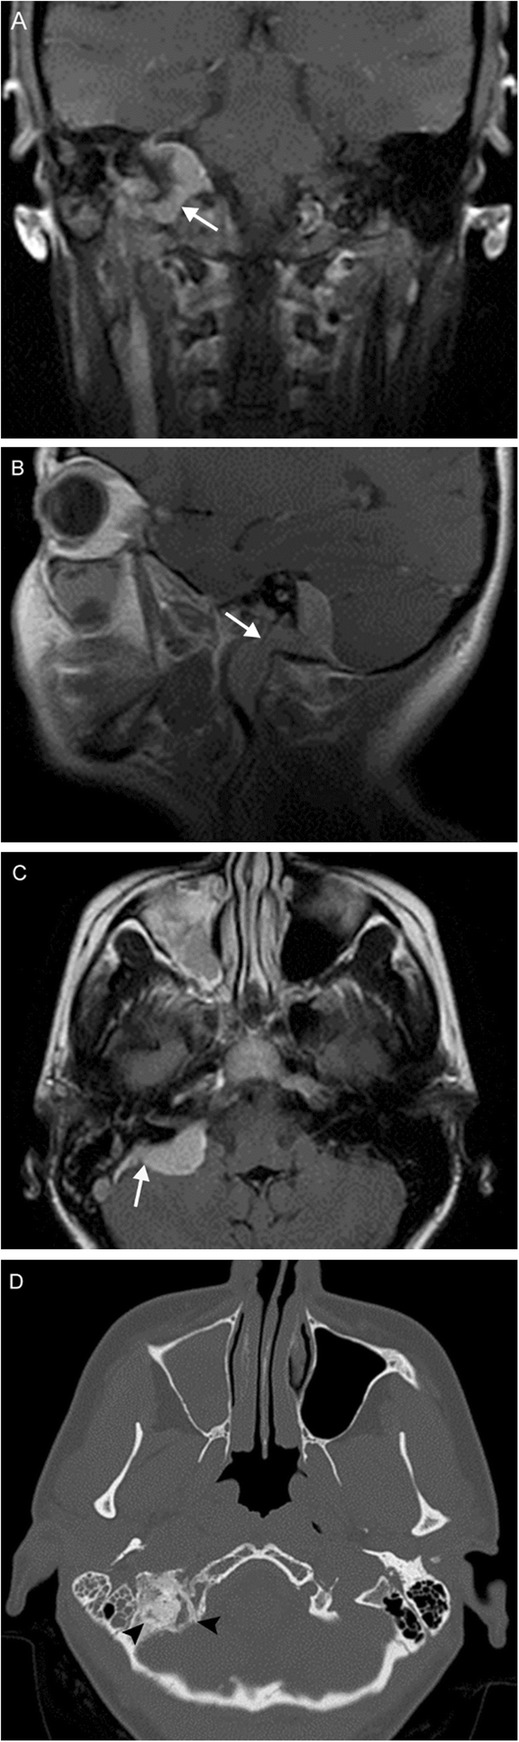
Meningioma. A 45-year-old woman initially presenting with right sensorineural loss, followed by headache, dysphagia, dysphonia, imbalance, and vasovagal syncope. Coronal (a), sagittal (b), and axial (c) post-contrast T1-weighted MR images reveal a dural-based mass at the right cerebellopontine angle (white arrows) extending into the internal auditory canal, as well as through the jugular foramen into the carotid space. Axial contrast-enhanced CT (d) reveals diffuse hyperostosis of the right temporal bone with opacification of the mastoid air cells (black arrowheads). Overall, findings are consistent with a meningioma. Incidental severe right maxillary sinus mucosal thickening is also seen
